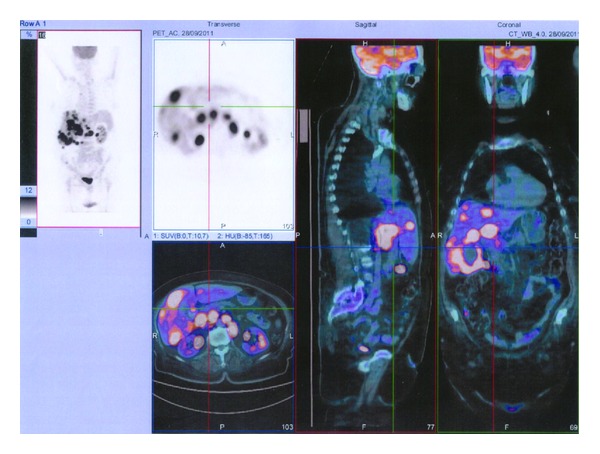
Restaging PET/computed tomography.
